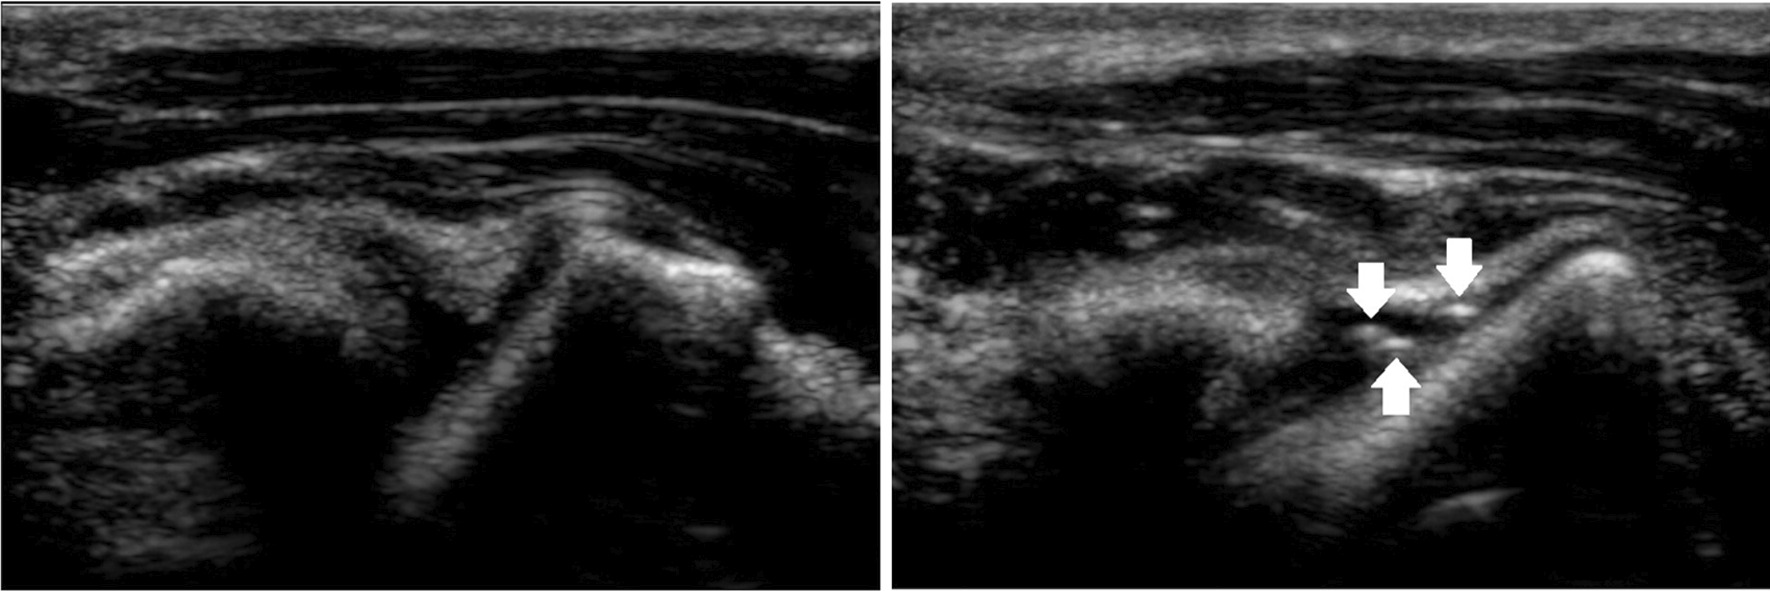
Ultrasound images before and after cavitation in a trapeziometacarpal joint Reproduced from Jones et al. [32]
